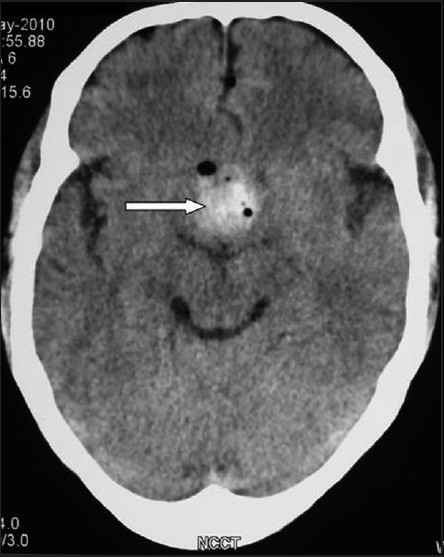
A computed tomographic scan of the head showing a large haematoma in the sellar region (bold white arrow)
